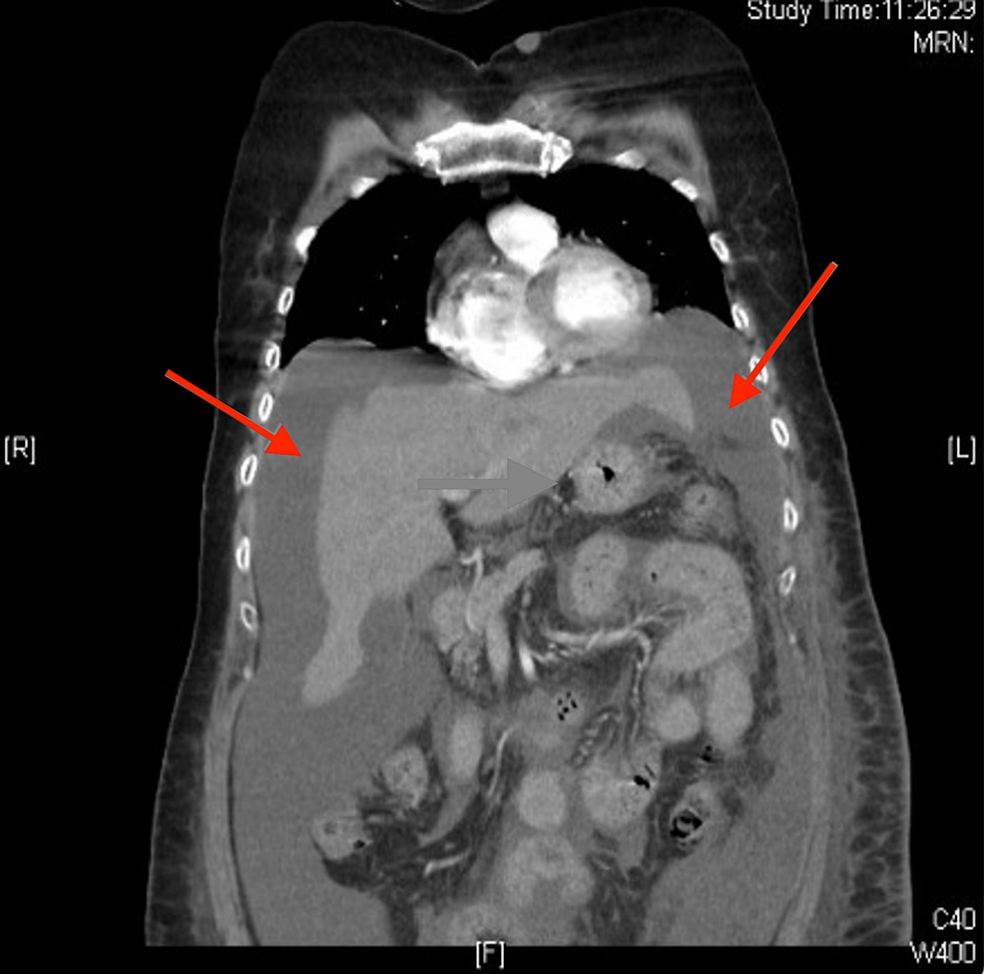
Coronal CT section showing abundant perihepatic, perisplenic and around bowel loops fluid. Red arrows point perihepatic, perisplenic and around bowel loops fluid.
